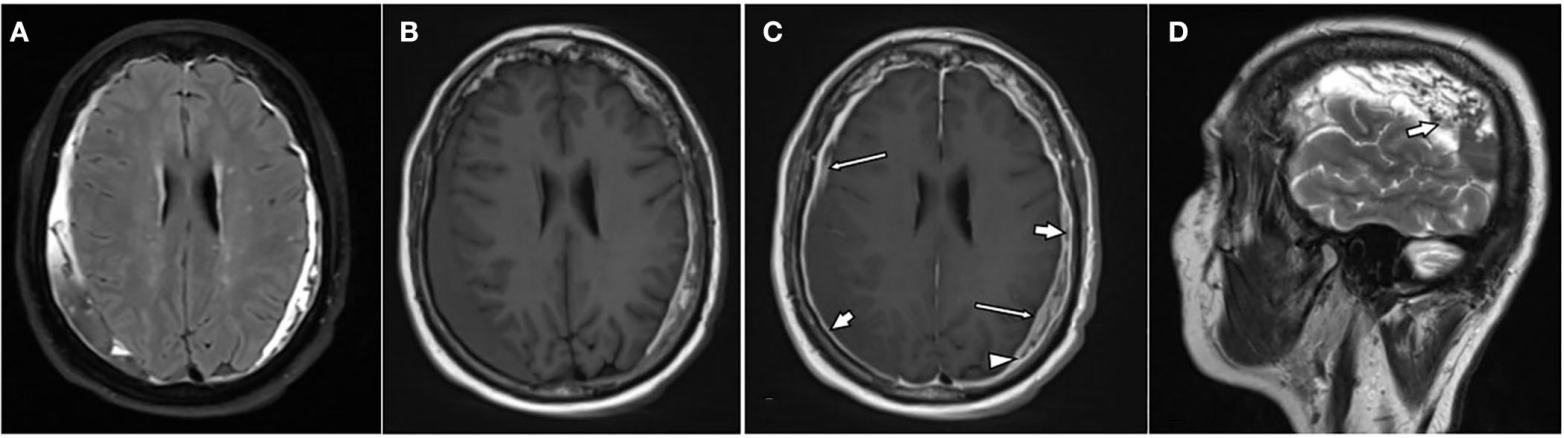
A 76-year-old female patient with bilateral chronic subdural hematomas (cSDHs). Axial-T2-FLAIR image (A), axial T1w images pre (B), post (C), and contrast administration as well as a sagittal T2w over the right hematoma (D). The hematomas are of mixed signal intensity on T2-FLAIR (A), T1w (B), as well as T2w images [(D): arrow]. There is enhancement of the inner [(C): thin arrows] and outer membrane [(C): arrows] as well as spandrel-like contrast enhancement at the borders of the hematoma [(C): arrowhead].
